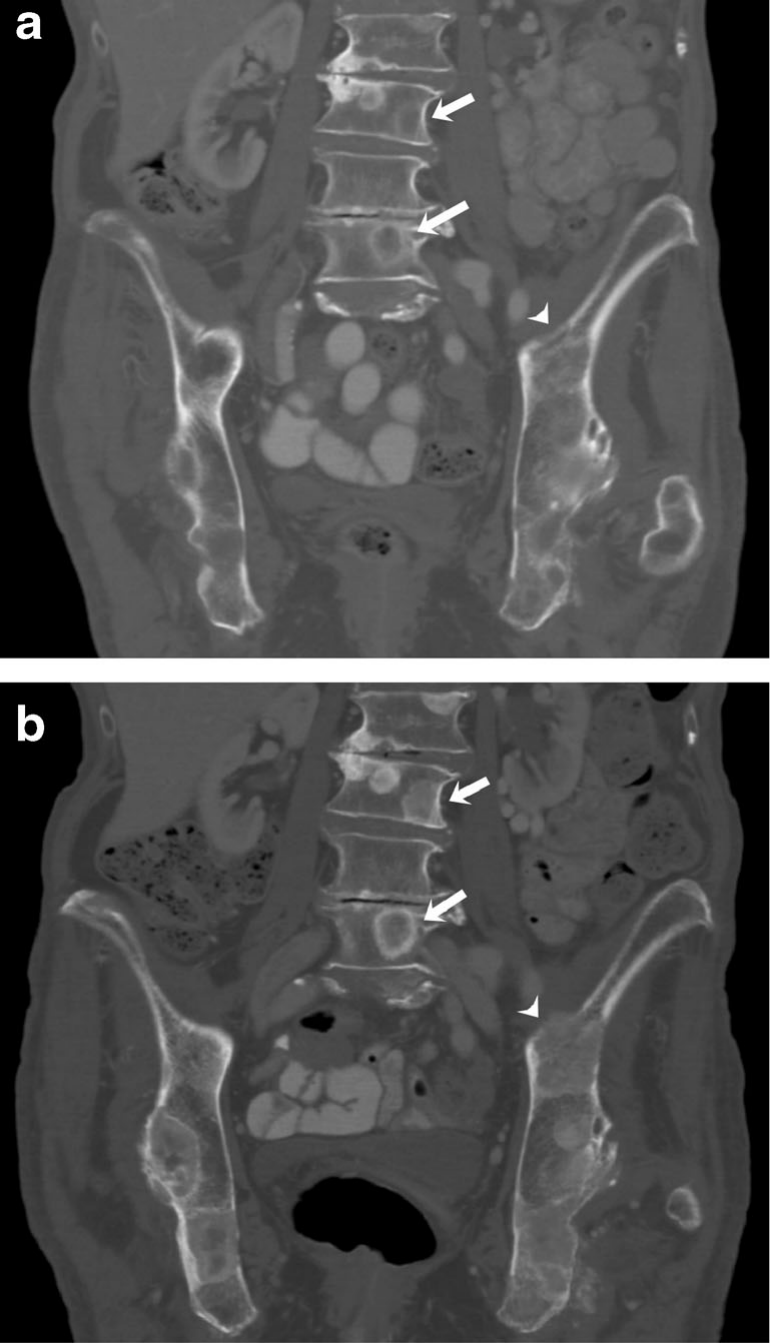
82-year-old woman with estrogen and progesterone receptor-positive breast cancer with bony metastases being treated with systemic chemotherapy. a Coronal pretreatment CT image of the lumbar spine and pelvis in bone window settings shows a predominantly lytic lesion involving L3 and L5 vertebrae (*arrows*) and left iliac bone (*arrowhead*). b Coronal CT image of the lumbar spine and pelvis after 4 months of therapy shows progressive sclerotic changes involving L3 and L5 vertebrae (*arrow*) and left iliac (*arrowhead*) lesions representative of treatment effect. This response is in agreement with patient’s improved clinical status and tumour marker levels
